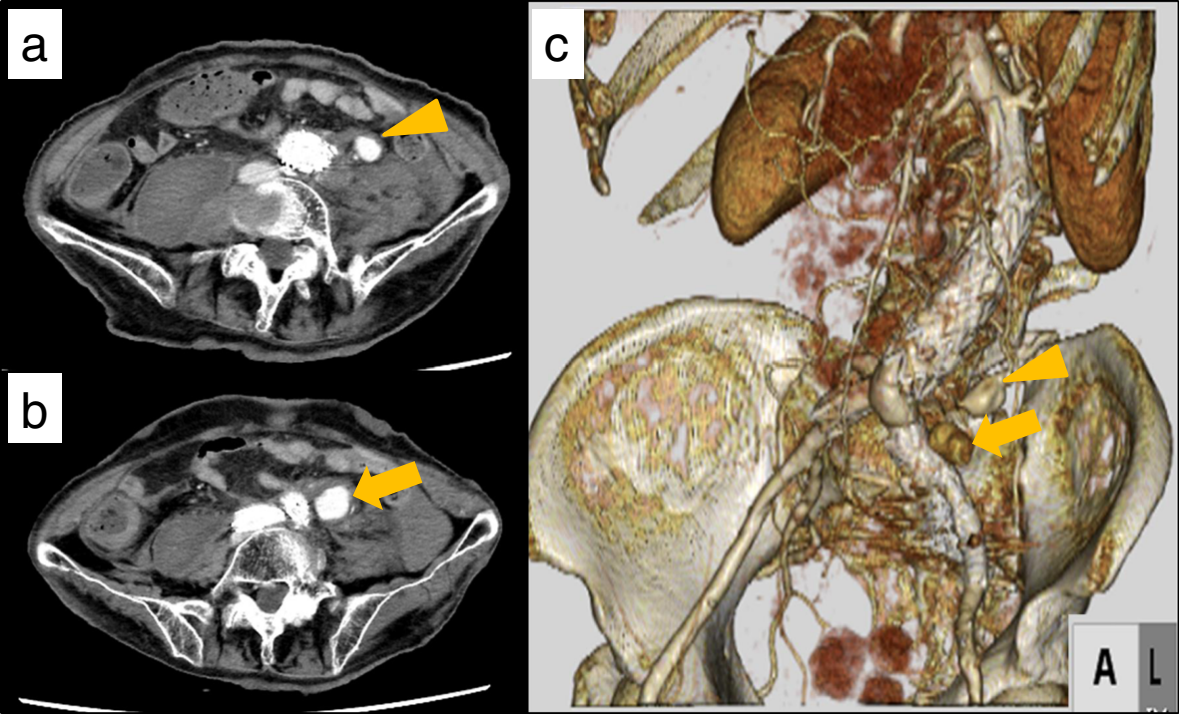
Radiographic images of the left colic artery aneurysm prior to the patient’s re-operation. a, b A CT scan of the abdomen and pelvis demonstrated the distal (a) (orange arrowhead) and proximal (b) portions of the left colic artery aneurysm (orange arrow). c A 3D-CT scan reconstruction of the abdomen and pelvis demonstrated the two locations of the left colic artery aneurysms (orange arrowhead and orange arrow)
